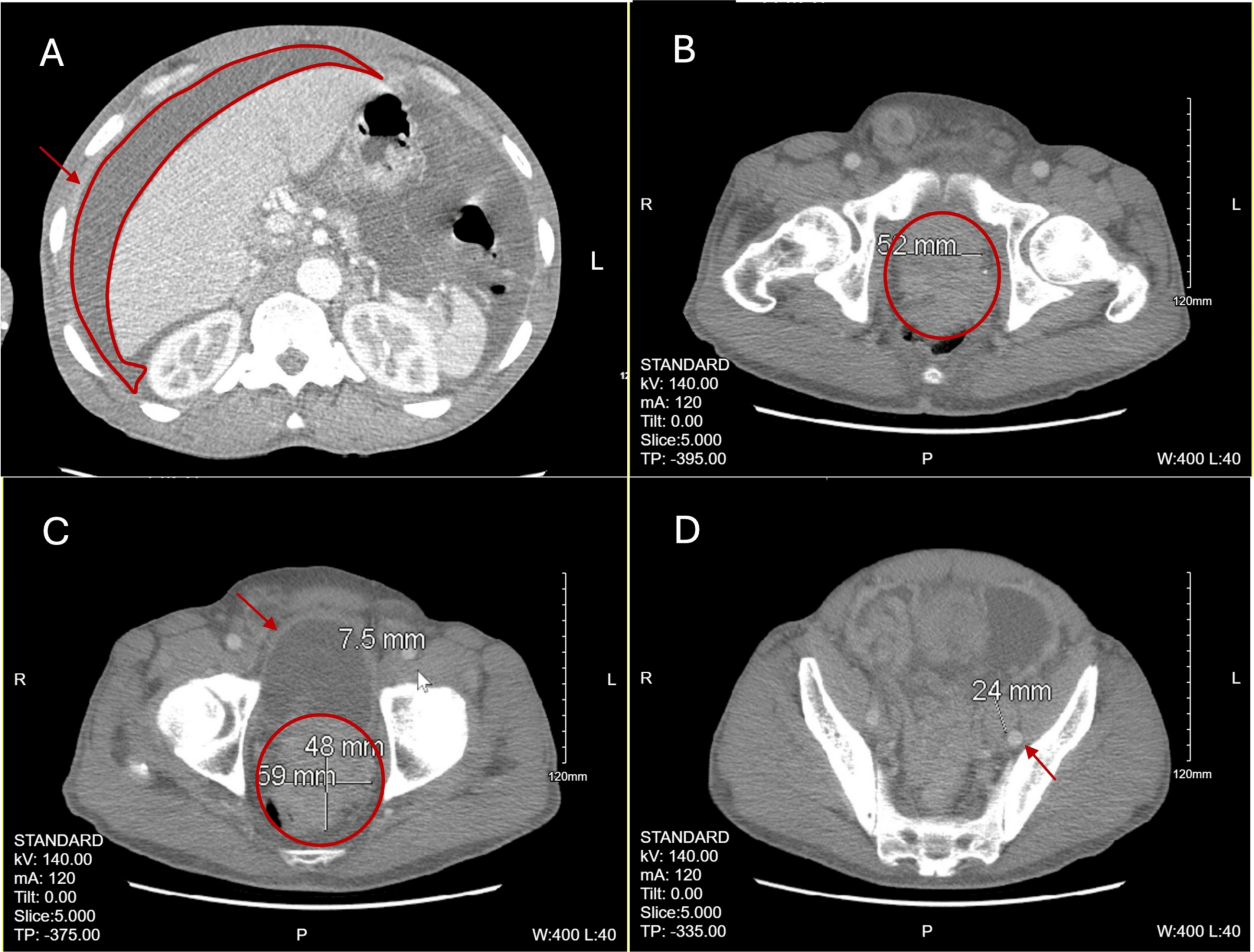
Patient’s CT abdomen and pelvis with IV contrast. (A) Massive ascites (circle) with enhancement of the peritoneum (arrow), (B) enlarged prostate gland (circle), (C) continuous soft tissue mass extending from the prostate gland, involving the rectum (circle), thickened bladder wall (arrow), (D) significant pelvic lymphadenopathy (arrow).
